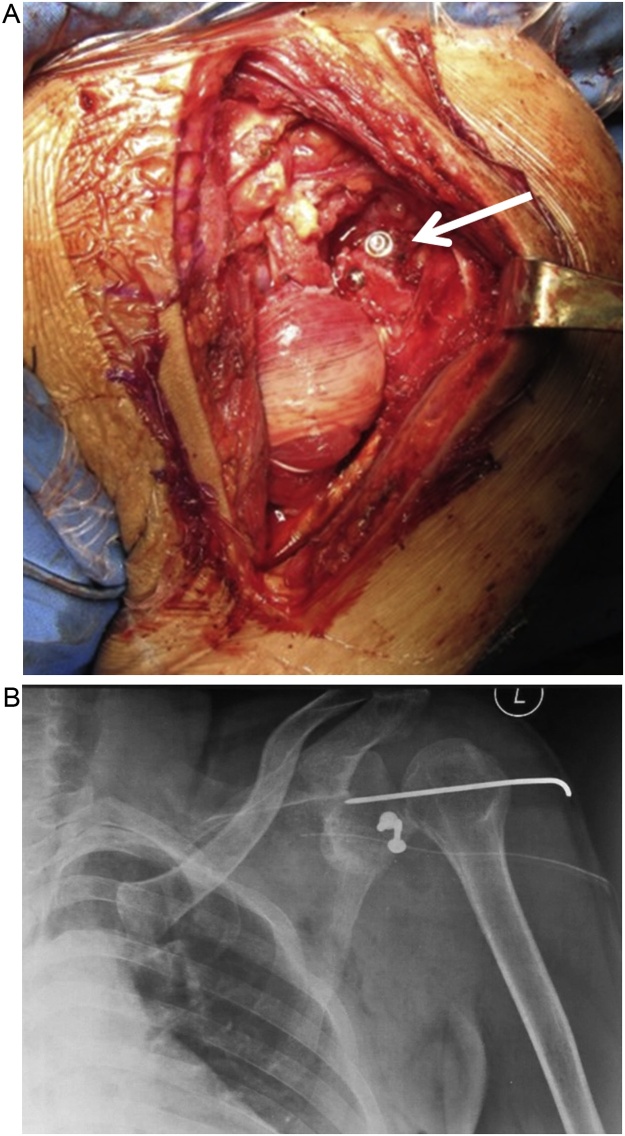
(a) Latarjet procedure: osteotomized coracoid process has been attached to antero-posterior part of the glenoid rim (pointed by arrow). (b) Post-operative X-ray antero-posterior view of the left shoulder showing a reduce glenohumeral joint with fixation of Kirschner wire and 2 screws, in accordance with Latarjet procedure.
